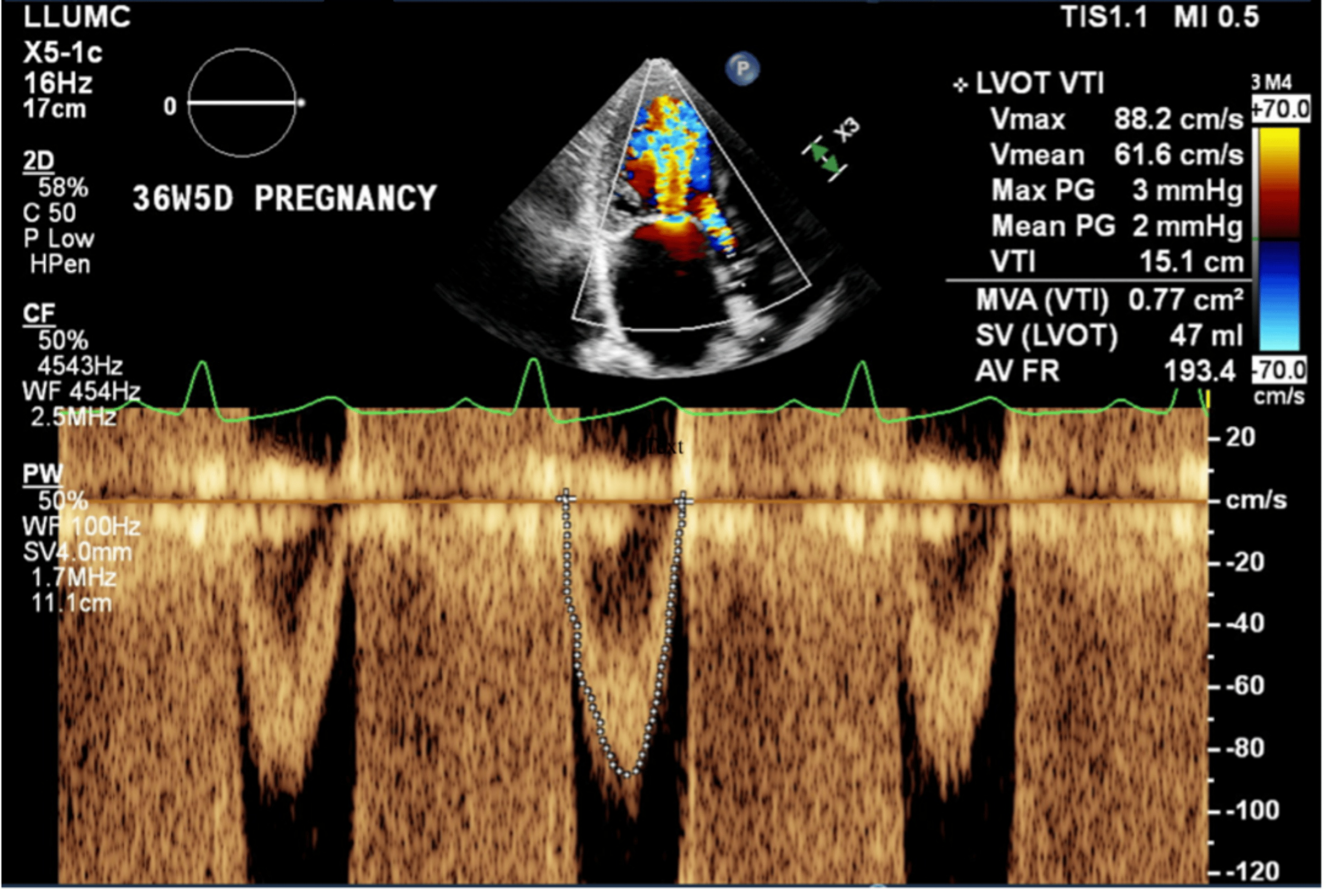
Doppler echocardiographic assessment of the left ventricular outflow and mitral valve area at 36 weeks' gestation Continuous-wave Doppler echocardiography demonstrating the left ventricular outflow tract (LVOT) velocity-time integral (VTI) in a 36-week pregnant patient with severe rheumatic mitral stenosis. The calculated MVA via VTI is 0.77 cm², consistent with severe stenosis. LVOT VTI is 15.1 cm, with a stroke volume of 47 mL and an AV FR of 193.4 mL/s. Abbreviations: LVOT = left ventricular outflow tract; VTI = velocity-time integral; MVA = mitral valve area; SV = stroke volume; AV FR = aortic valve flow rate; PG = pressure gradient
